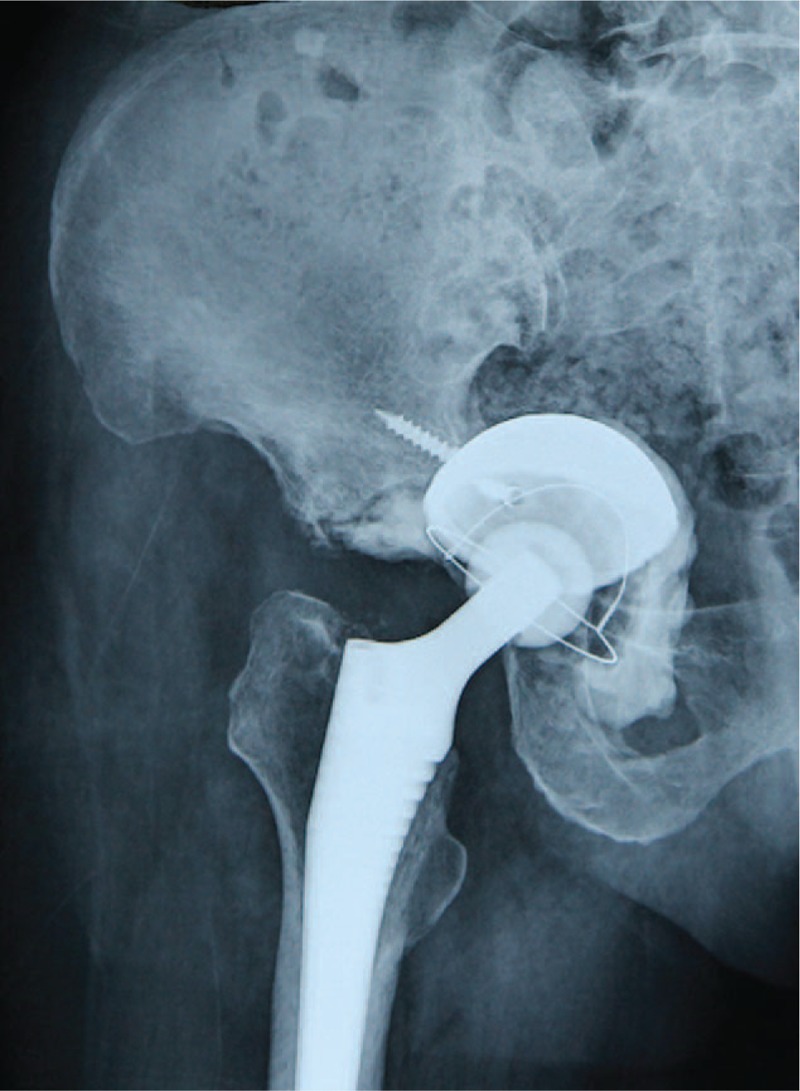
Postoperative anteroposterior radiographs of the patient after polyethylene-liner cementation revealing favorable state of the prosthesis.
